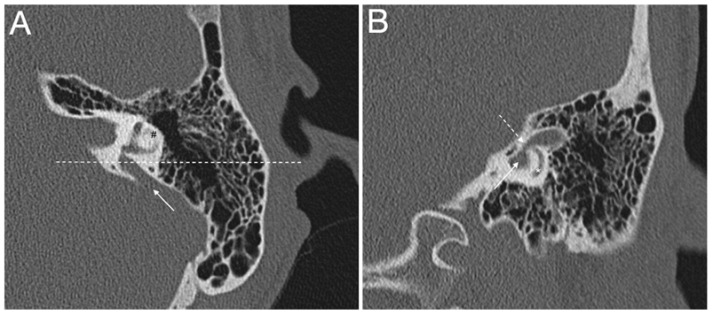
(A,B) Computed tomography of a left temporal bone of a 12-year-old female patient with bilateral enlarged vestibular aqueduct and congenital moderate (left ear) to profound (right ear) hearing loss. Section A: axial plane; arrow is the enlarged vestibular aqueduct; #, lateral semicircular canal; dotted line is the plane of section B. Section B: coronal plane; continuous arrow is the enlarged vestibular aqueduct; dotted arrow is the isthmus of the enlarged vestibular aqueduct; *, posterior semicircular canal. The patient harbors biallelic pathogenic variants (c.1301C > A; p.A434D and c.1730T > C; p.V577A) in the *SLC26A4* gene, which were described in [43].
